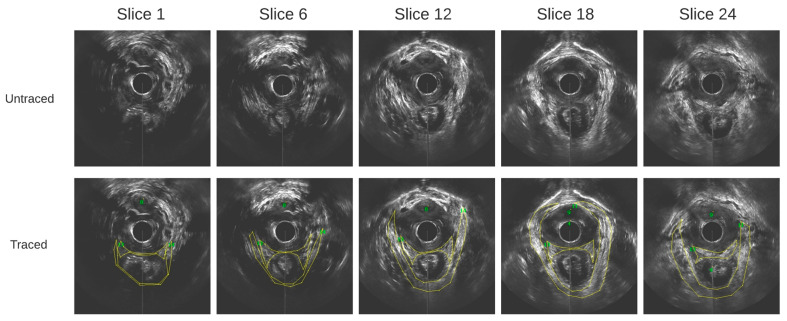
Axial ultrasound images extracted from the 3D EVUS data from one healthy participant at varying slice depths, and their corresponding traces.
